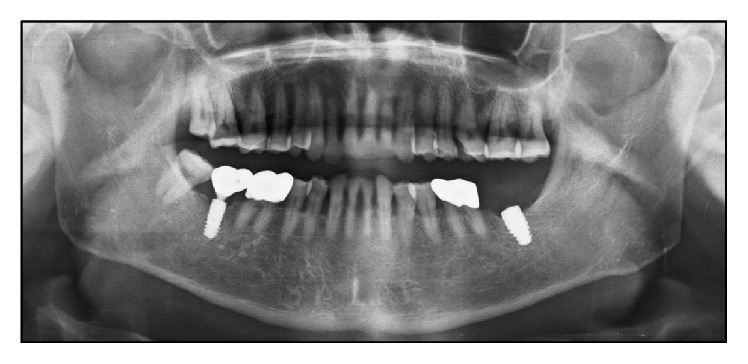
Preoperative radiographic view (panoramic X-ray image). Implant of #37 area shows the broken abutment screw off the implant fixture inside.
